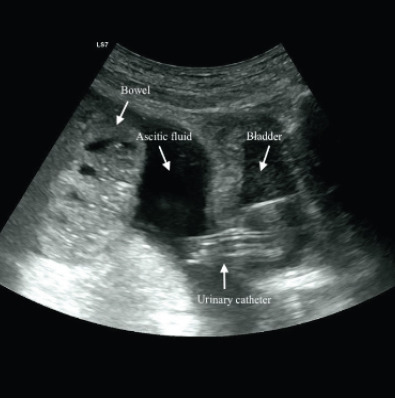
Abdominal ultrasonography confirming extrusion of the urinary catheter through the superior surface of the bladder associated with traumatic rupture.
